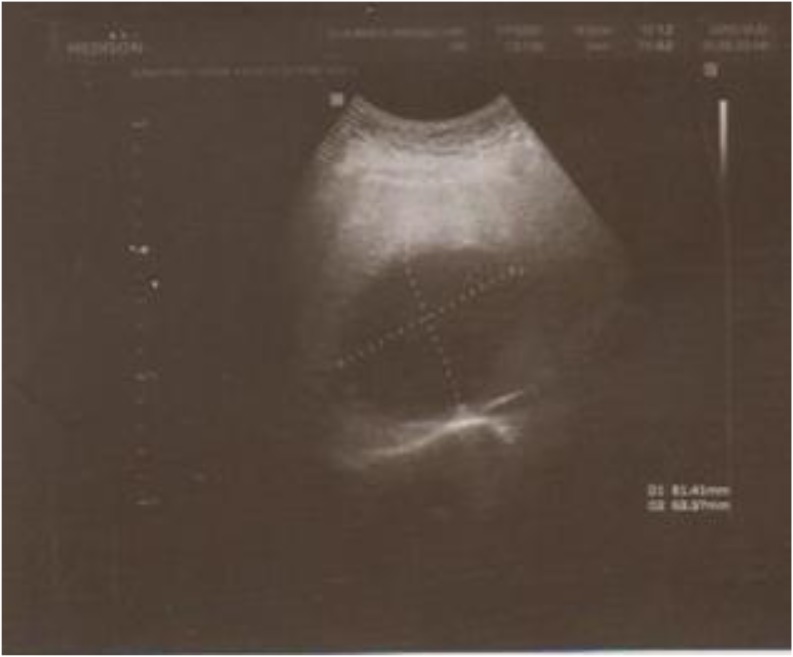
Abdominal ultrasound shows large hydatid cyst compressing inferior vena cava
